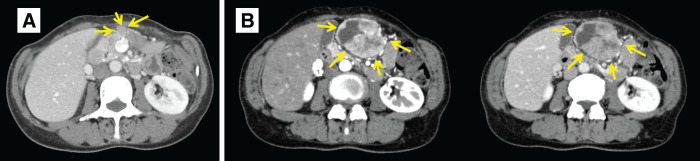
Preoperative CT images. (A) CT scan conducted at a previous hospital. It showed a 9-mm mass on the greater curvature side of the gastric antrum (arrows). (B) CT scan conducted at our hospital. A 55-mm hypervascular mass with internal necrosis and a clear border was observed at the sub-umbilical level (arrows). The tumor demonstrated early enhancement in the arterial phase (left image), with a decrease in the contrast effect in the late phase (right image).
